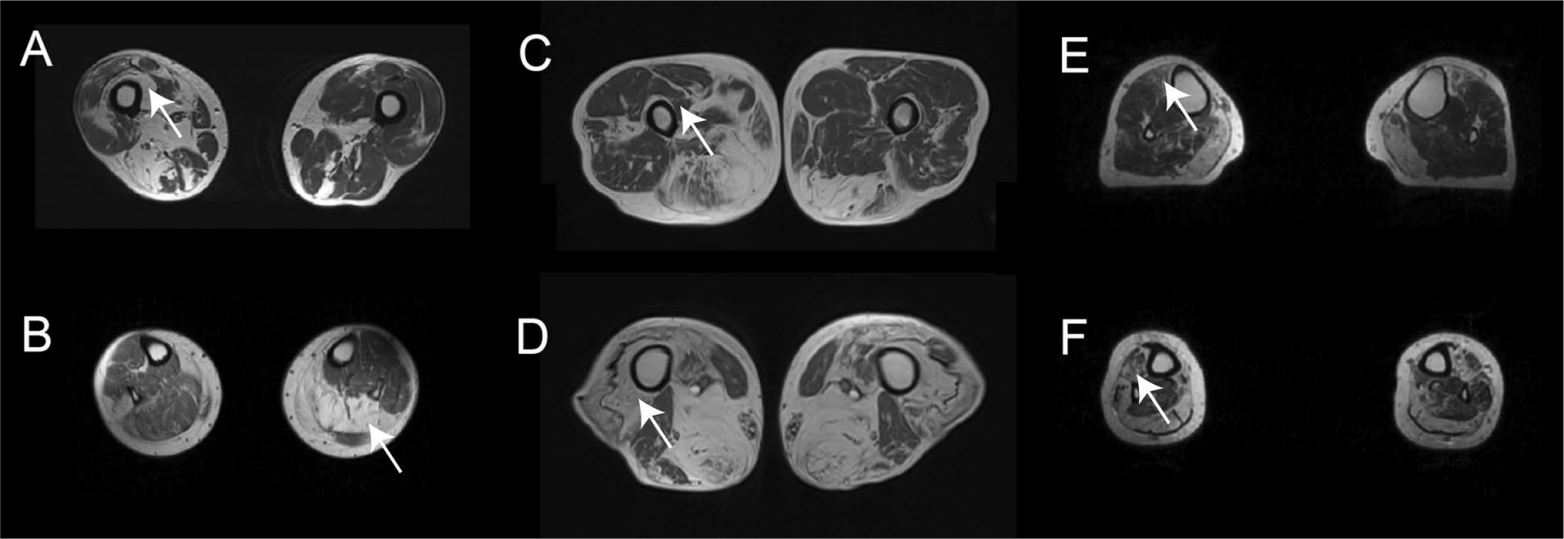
Asymmetric and distal to proximal gradient of fat replacement. A and B show MRI of two patients with asymmetric fat replacement in the vastus intermedius (arrow in A) and the soleus (arrow in B) muscles. C and D show a distal to proximal gradient of fat replacement in the *vastus intermedius* muscle (arrow). E and F show a distal to proximal gradient of fat replacement in the *tibialis anterior* muscle (arrow)
